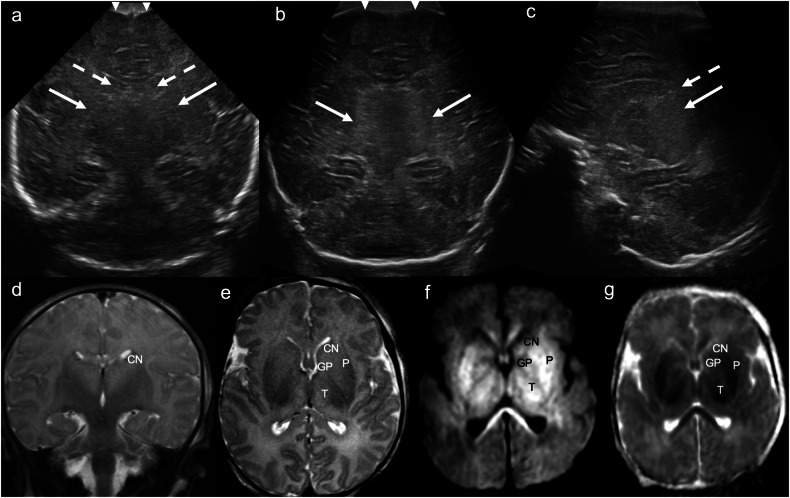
Hypoxic ischaemic injury. Day 1 ultrasound in a term neonate with cord prolapse, poor APGAR scores, and seizures at birth. a, b Coronal images demonstrate “slit-like” lateral ventricular frontal horns (dashed arrows), diffuse effacement of extra-axial CSF spaces (white arrowheads), and bilateral, symmetrical increased echogenicity of the basal ganglia (a) and thalami (b) (solid arrows). c Parasagittal image demonstrates an effaced lateral ventricle (dashed arrow) and increased echogenicity and swelling of the thalamus (solid arrow). d Coronal and (e) axial T2WI, (f) axial trace DWI and (g) ADC map demonstrate corresponding bilateral, symmetrical T2 hyperintensity and swelling (d, e) and restricted diffusion (high signal on DWI, low signal on corresponding ADC map) (f, g) involving the caudate nuclei, lentiform nuclei (globi pallidi and putamina), and thalami
